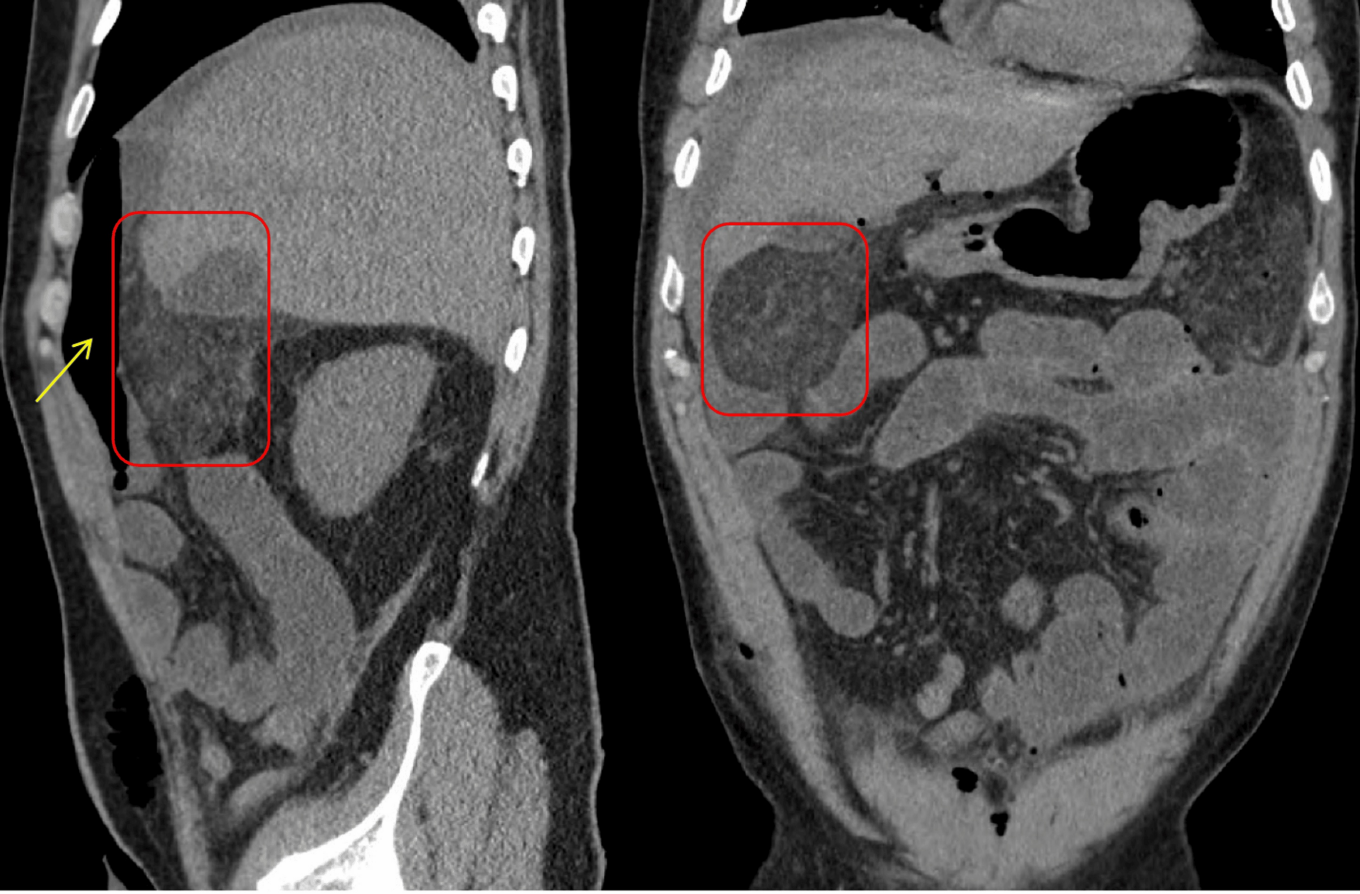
CT scans of the abdomen and pelvis without contrast presented in sagittal (left) and coronal (right) views. The images reveal mesenteric stranding and the presence of pneumoperitoneum (highlighted by the yellow arrow) in the right upper quadrant. The red boxes delineate the areas of mesenteric stranding, suggesting possible postoperative changes or complications.
